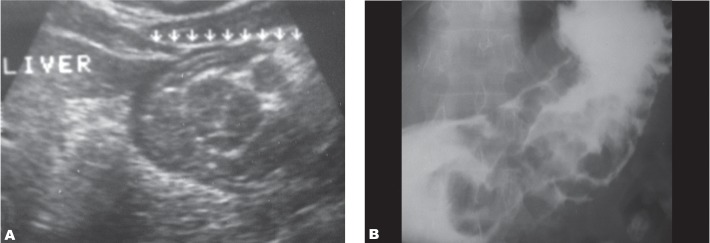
Abdominal ultrasound (A) and barium meal (B) images demonstrating giant hypertrophy of the gastric mucosal folds in a 52-year-old man, probably related to chronic alcohol consumption.
